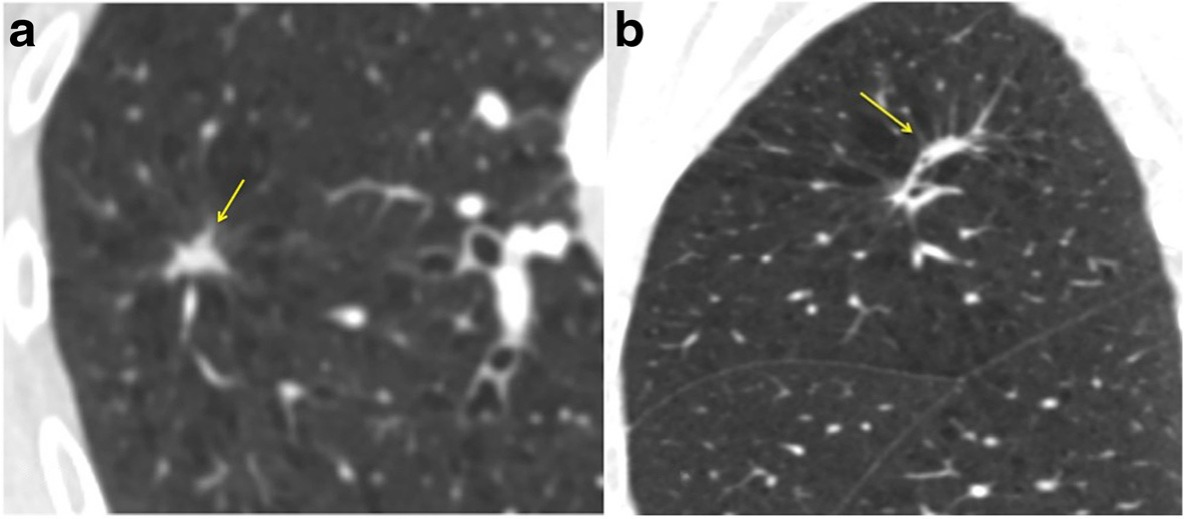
a Axial CT shows a possible spiculated 10x8 mm nodule (arrow). b On the Coronal reformat the lesion is ‘flat’ and likely represents focal scarring/linear atelectasis and is not suitable for DCE-CT (arrow)
